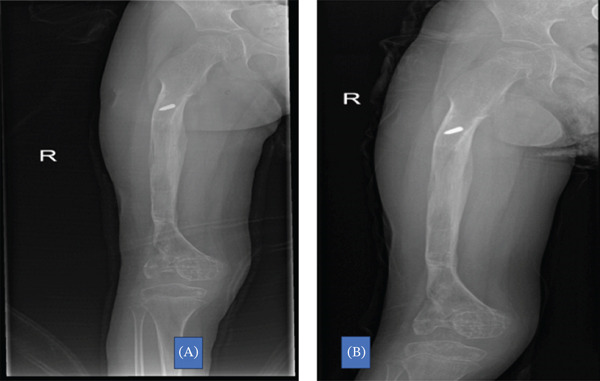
Radiograph (after frame removal) at (A) 7 months and (B) 8 months confirming solid regenerate consolidation across both corticotomy sites and restoration of femoral length; the knee joint line is horizontal and congruent.
